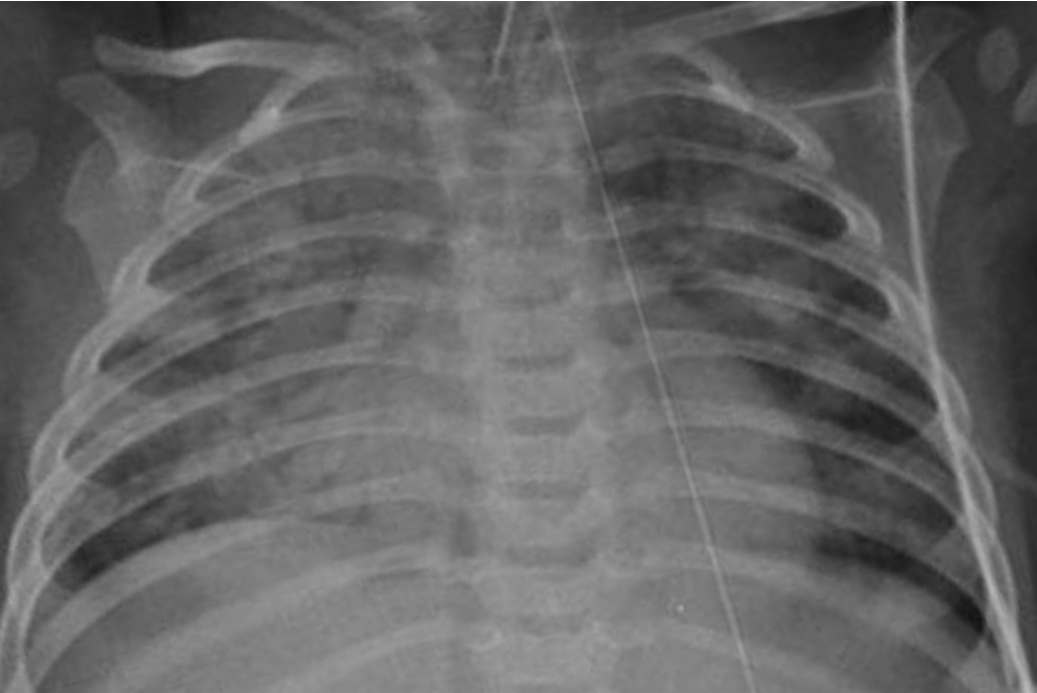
Chest X-ray of case 2.
